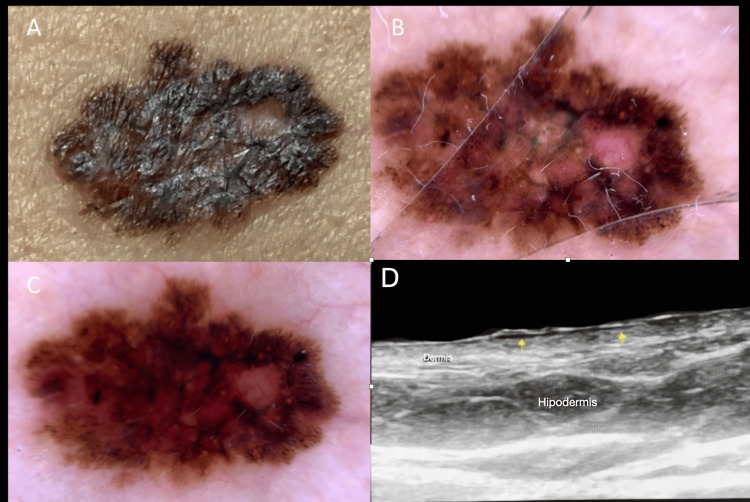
Dermoscopic and ultrasound features of a vertically growing melanoma. (A) Clinical examination shows a patchy, pigmented lesion. (B, C) Dermoscopy reveals a poorly defined, melanocytic lesion with an irregular pigment network, white areas, and a small, unpigmented, raised area with telangiectasias on its surface, suggesting vertical growth. (D) High-resolution ultrasound images show yellow arrows pointing to a well-defined, hypoechoic area in the upper dermis, which elevates the surface skin and indicates vertical growth. This ultrasound guided the biopsy, which confirmed superficial spreading melanoma.
